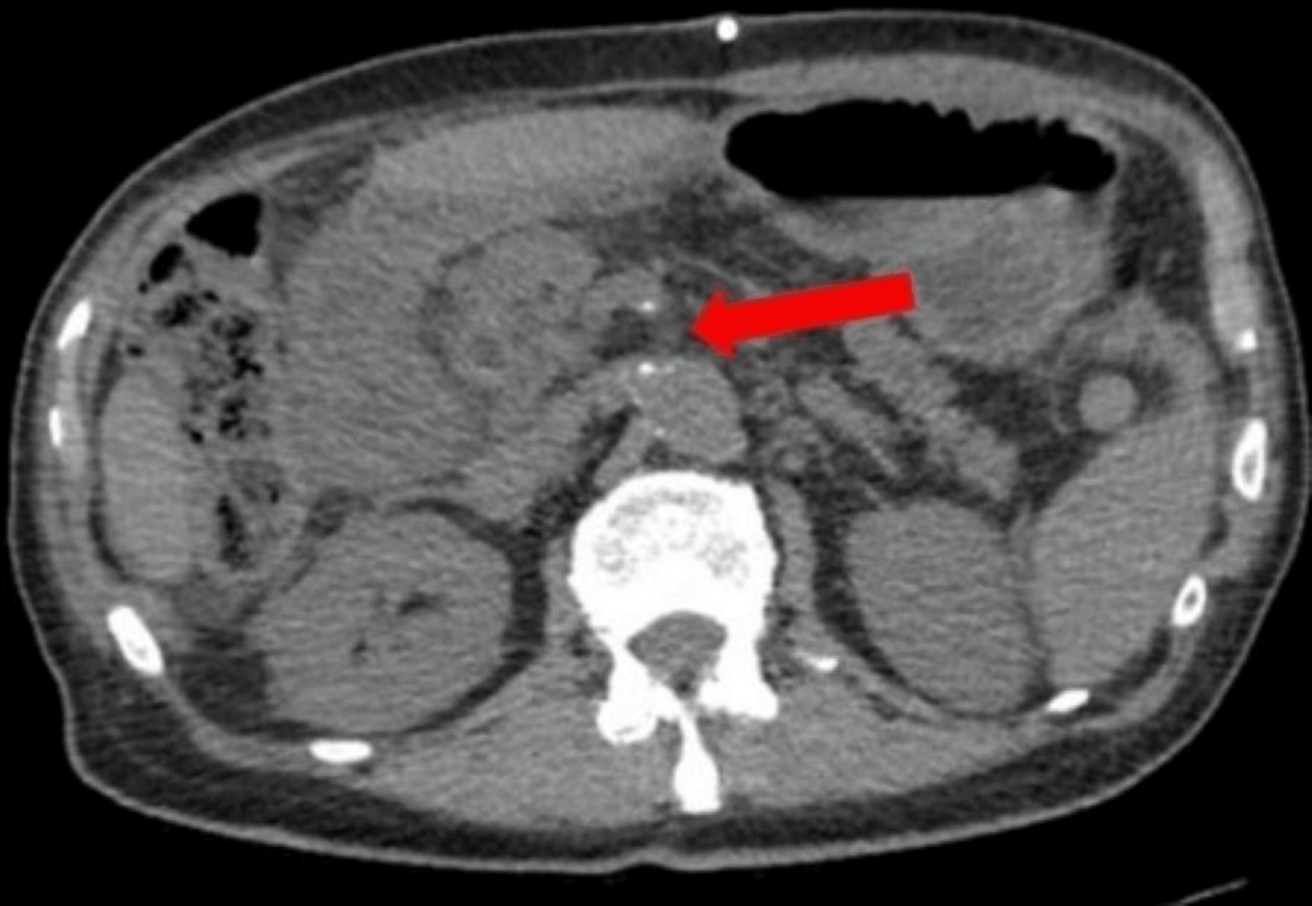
Repeat CT of the abdomen without contrast. Image showing a grossly similar appearance of the pancreatic tumor (arrow). Mildly prominent pancreatic lymph nodes and dilatation of the extrahepatic biliary, intrahepatic biliary, and pancreatic ducts can be visualized.
